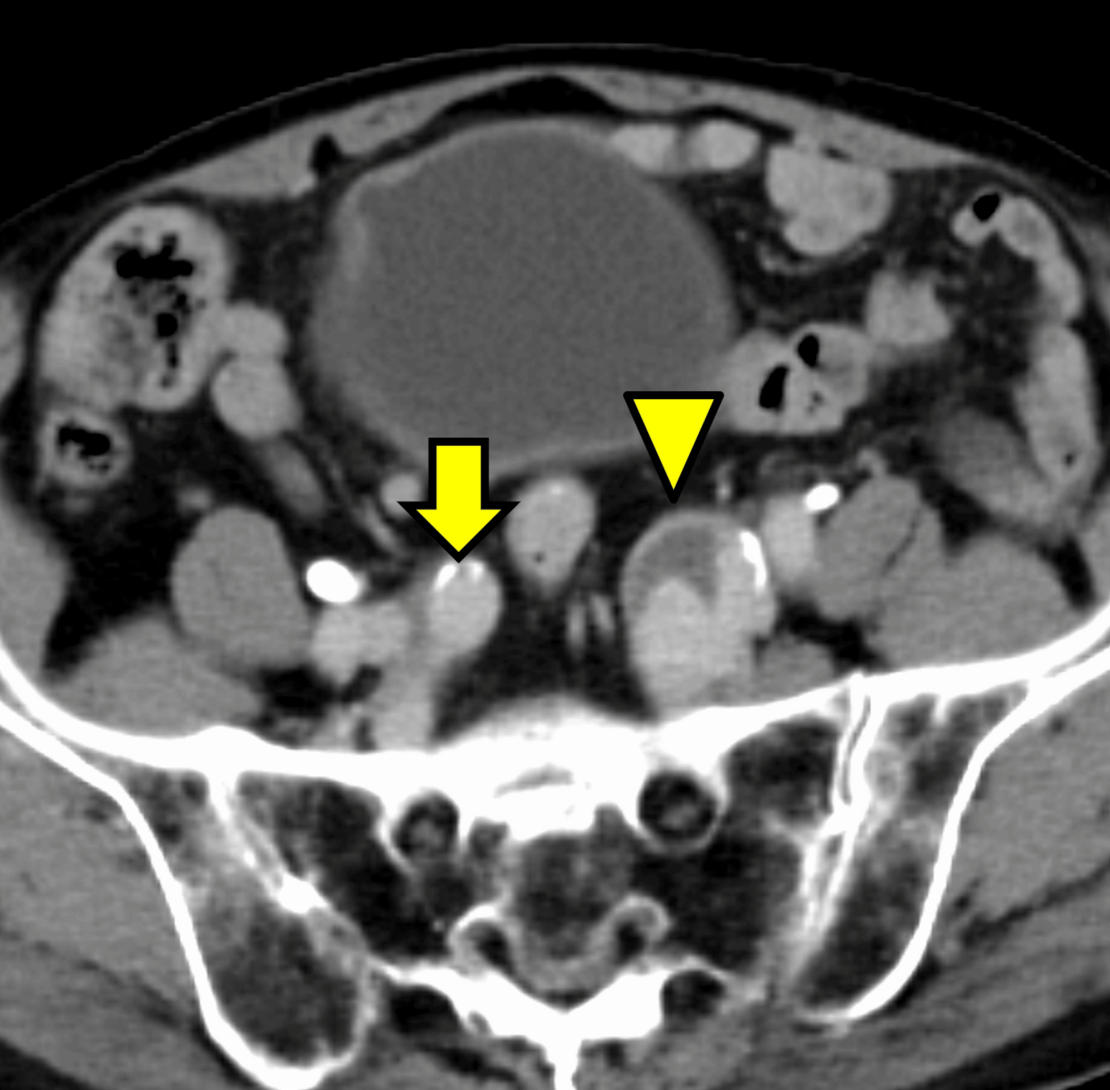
Contrast-enhanced CT 1-year post-treatment initiation. The soft tissue mass around the right internal iliac artery disappeared (yellow arrow), and a dissecting aneurysm appeared in the left internal iliac artery (yellow arrowhead).
